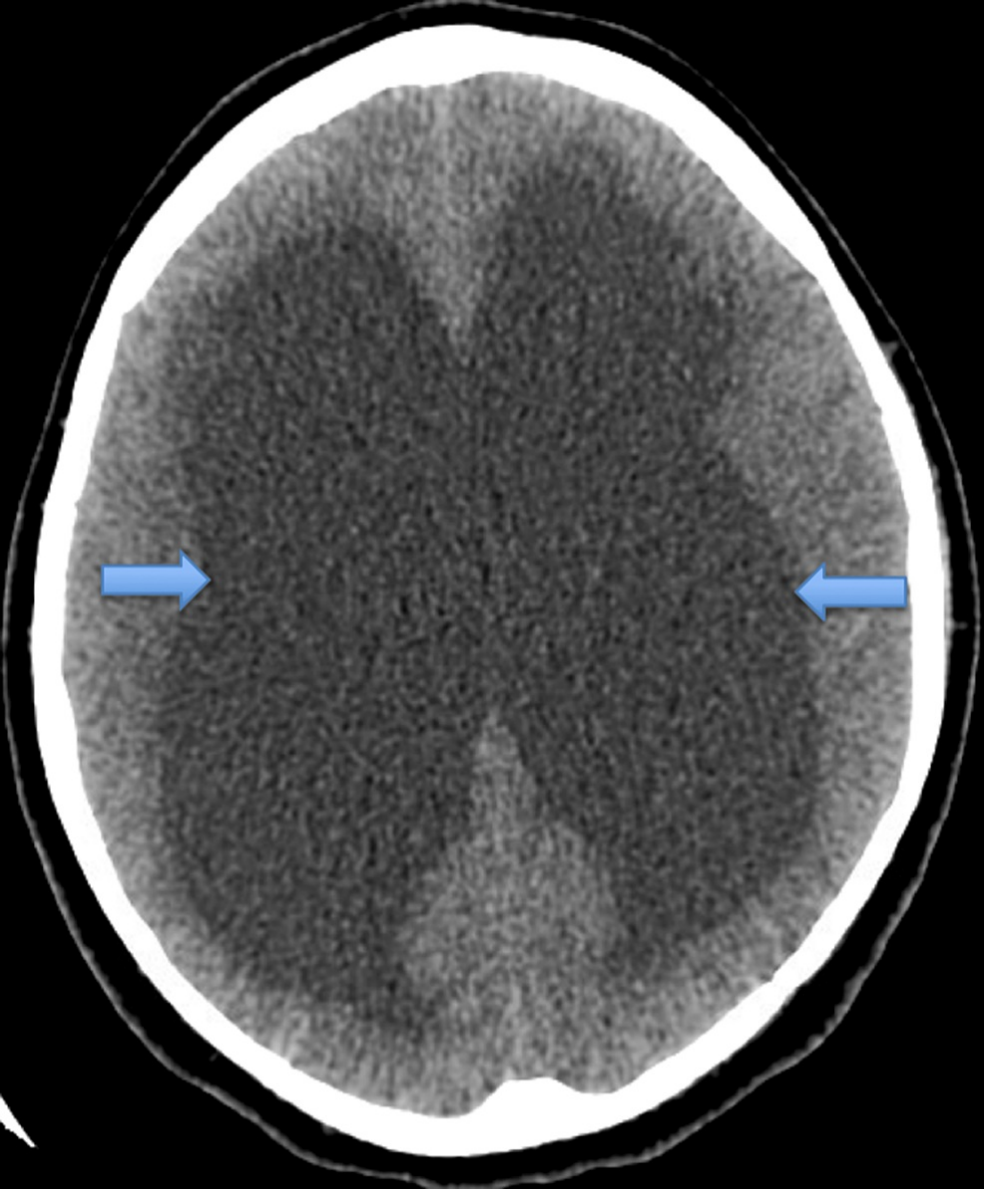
Computed tomography scan of the head without contrast showing acute hydrocephalus involving the lateral (indicated by arrows) and third ventricles, with trans-ependymal flow of CSF. CSF - cerebrospinal fluid
